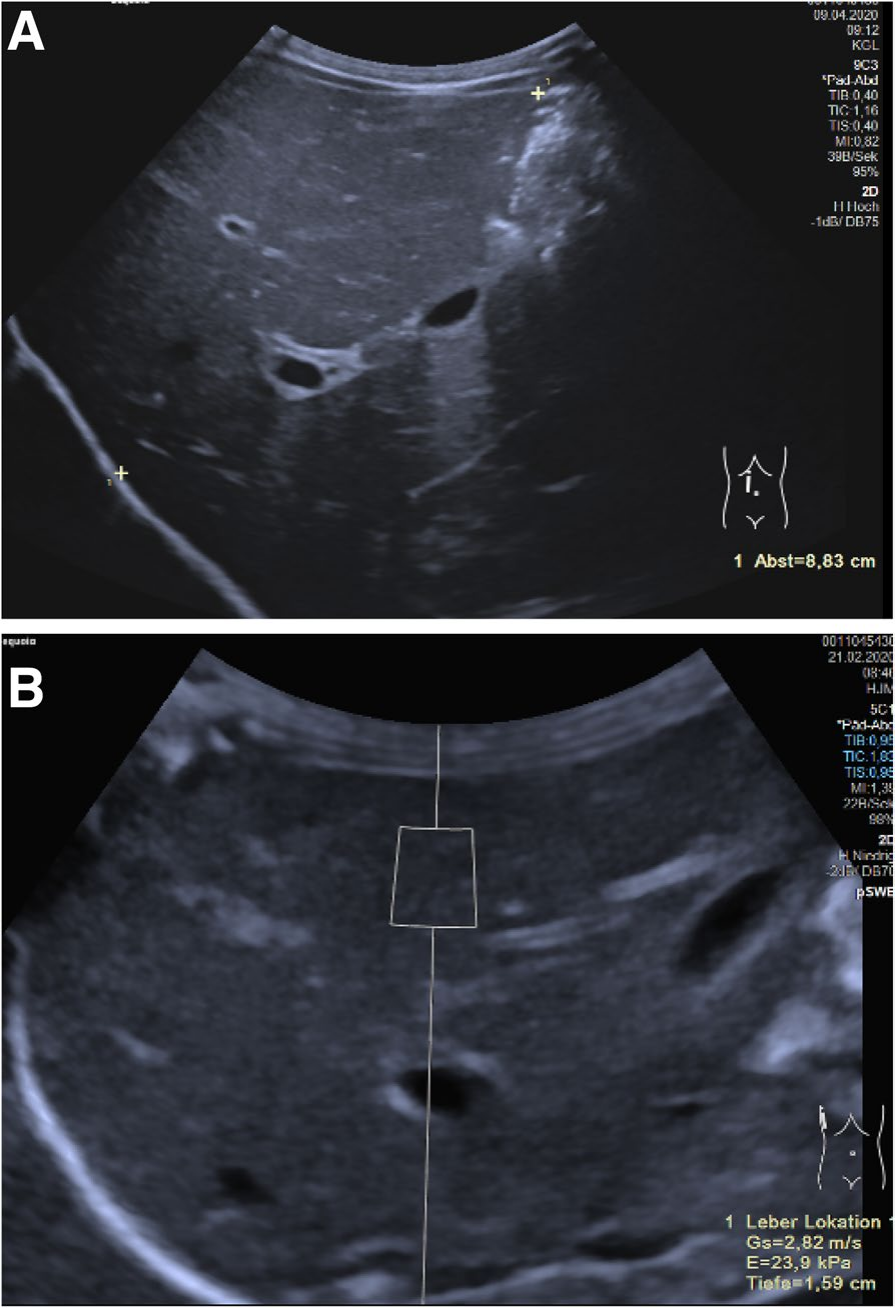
Four-month old male infant with hepatosplenomegaly and cholestasis. Following sonography and elastography biopsy indicated. The result showed Niemann-Pick disease type A associated with liver fibrosis and reduced synthetic capacity of the liver. A B-mode imaging of the liver in the midclavicular line corresponding to hepatomegaly (Sequoia, Siemens, 5C3 probe). B SWE measurement in the right liver lobe. Shear wave speed of 2.82 m/s is significantly increased and has to be assessed in terms of fibrosis (Sequoia, Siemens, 5C1 probe)
